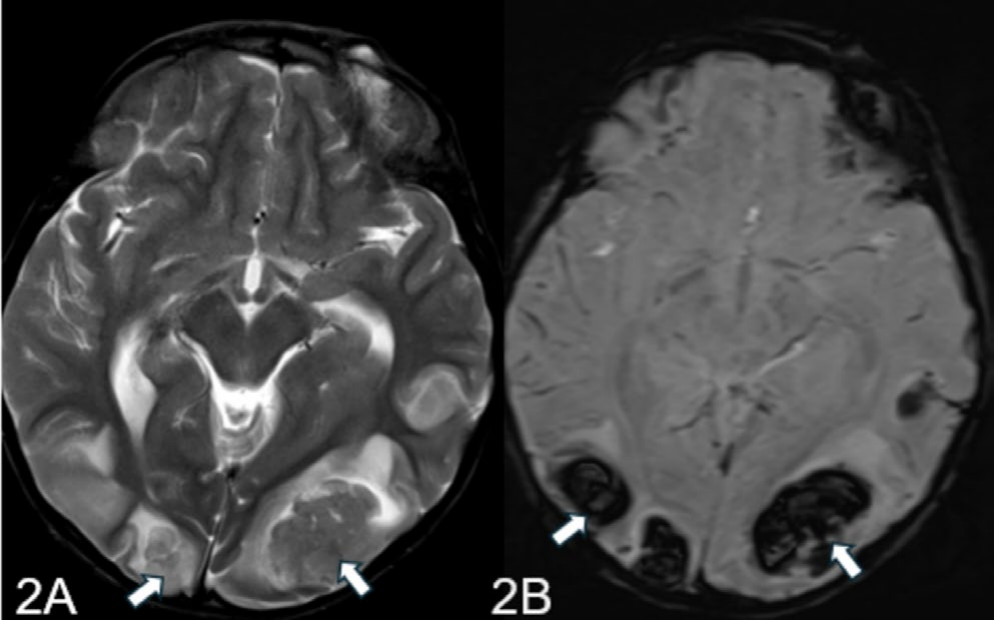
(A, B) MRI shows abnormal T2 signals in the bilateral occipital lobes showing signal drop out on SWI representing hemorrhage.
